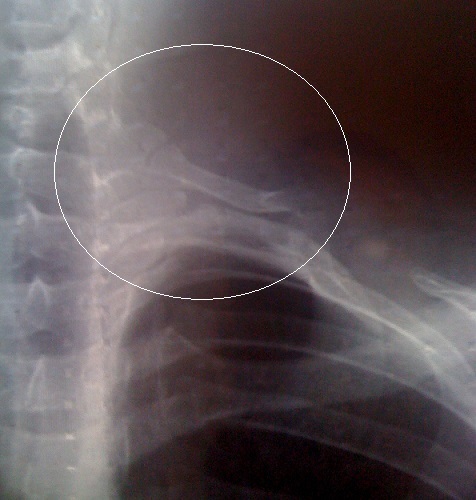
Radiographie du thorax montre la cote surnuméraire
